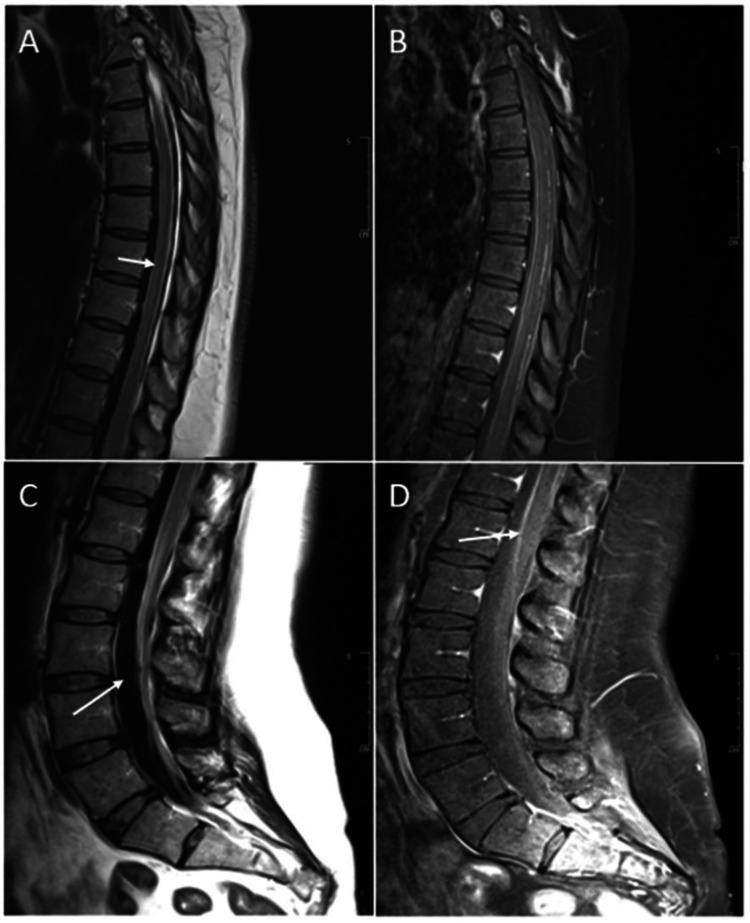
Sagittal MRI images from case two (A) T2 sequence of the patient's thoracic spine showing intradural hematoma (white arrow); (B) Post-contrast T1 sequence of the patient's thoracic spine showing leptomeningeal enhancement presumably due to irritation of the thoracic cord and nerve roots from the hemorrhage; (C) T2 sequence of the patient's lumbar spine showing intradural hematoma (white arrow); (D) Post-contrast T1 sequence of the patient's lumbar spine showing leptomeningeal enhancement around the conus (white arrow).
